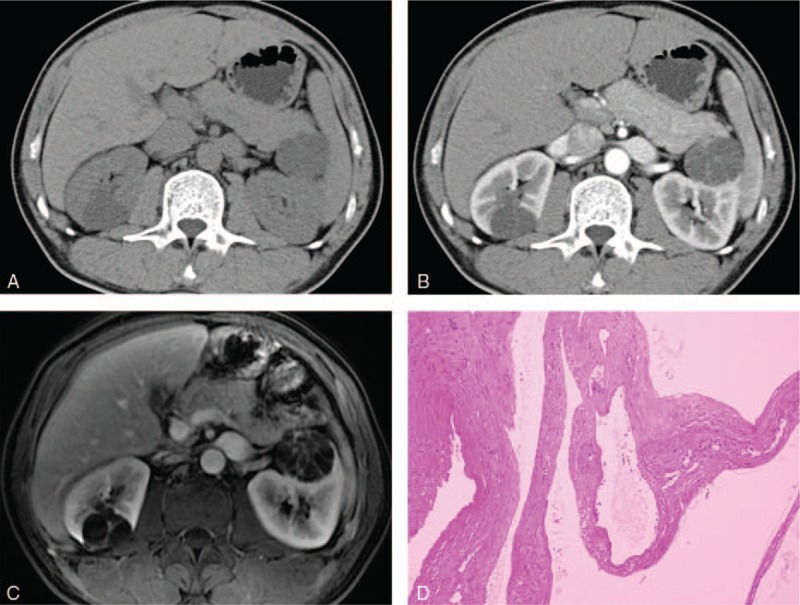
Images in a 45-year-old man with a cystic mass at the right and left kidney, respectively. (A, B) The right one at the axial contrast-enhanced CT scan demonstrated a minimally complex cystic mass that contains a few hairline-thin septa, suggesting a Bosniak category II cyst. The left one showed a complex cystic mass containing minimal thickened and enhancing wall and classified as a Bosniak category IIF cyst. (C) Gadolinium enhanced fat-suppressed T1-weighted MR image showed more septa than that on the CT scan, some of the septa are minimally thickened in the right lesion, and gross thickened septa depicted in the left lesion, caused the lesions to be upgraded to category IIF and III, respectively. (D) The left lesion was surgically removed and determined to be benign cyst. The right one underwent follow-up examinations for 36 months and showed no interval change. CT = computed tomography.
